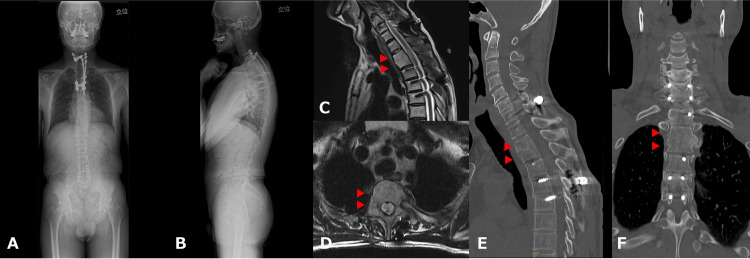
Imaging findings at one year postoperatively (A and B) Full-spine radiograph in a standing position showing good alignment maintenance. (C and D) Magnetic resonance imaging showing the disappearance of the abscess (arrowheads). (E and F) Computed tomography showing bone fusion of the affected vertebral bodies (T2 and T3) (arrowheads).
